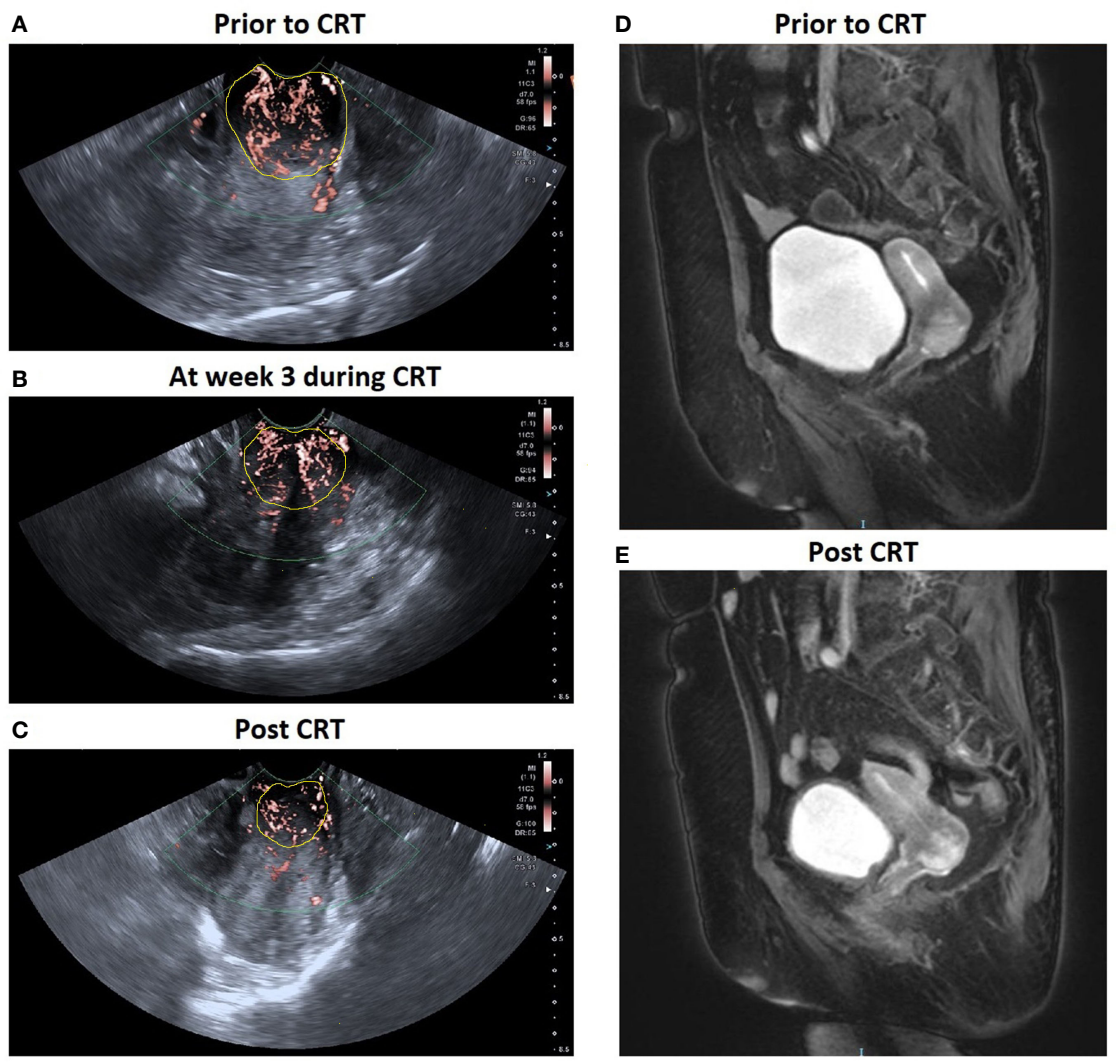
A patient with locally advanced cervical cancer (FIGO stage IIB) experienced complete response to CRT. SMI images show a significant decrease in VI in cervical cancer: (A) 0.398 prior to CRT; (B) 0.323 at week 3 during CRT; (C) 0.278 post CRT. Corresponding axial T2-weighted images exhibited a significant decrease in the maximal diameter of tumor: (D) 3.5 cm at pre-therapy e and (E) 1.8 cm post therapy.
